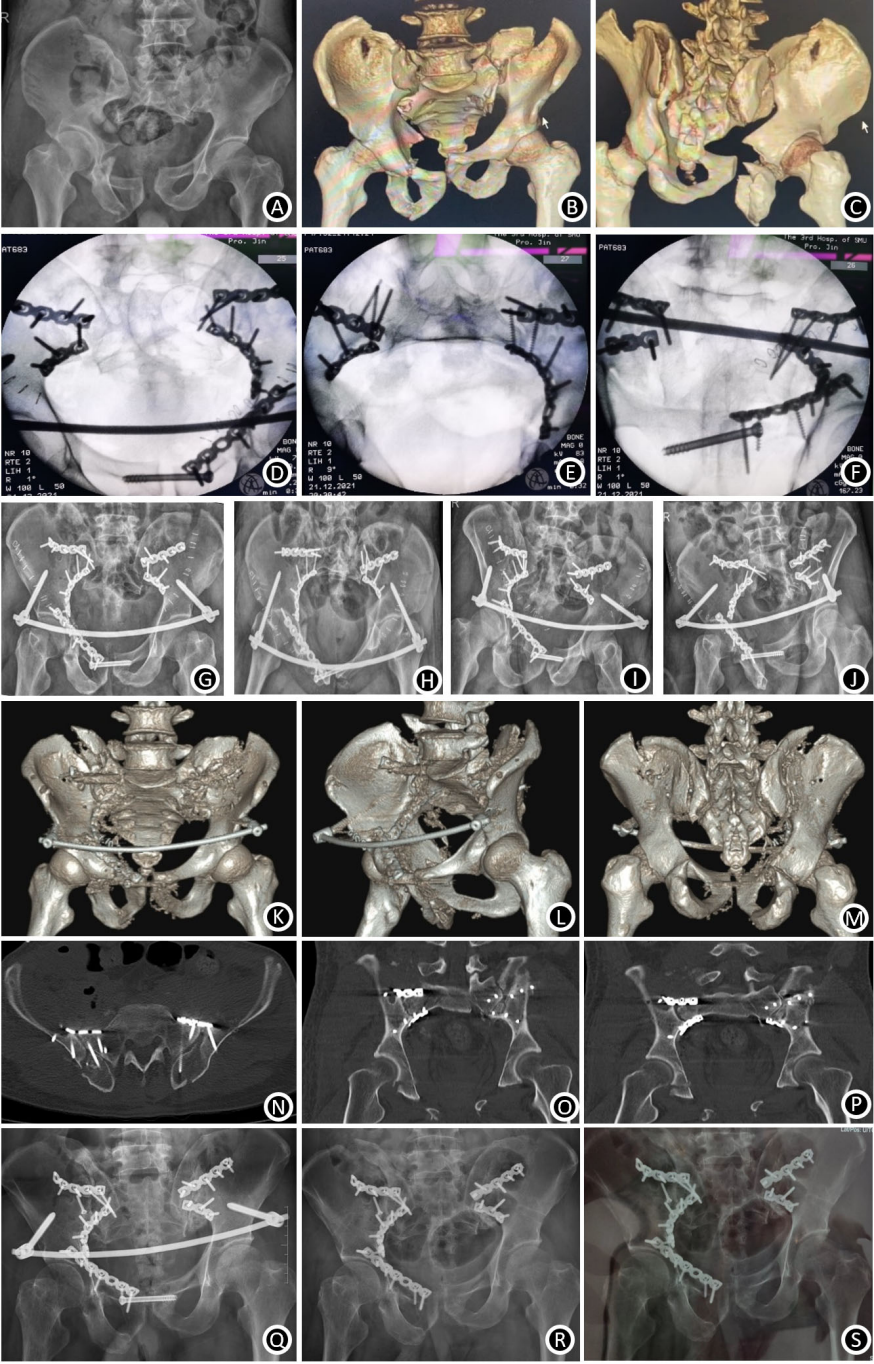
(A–C) Preoperative AP view and 3‐D reconstruction of CT data showed bilateral crescent‐shaped fractures of the posterior pelvic ring, anterior dislocation of the right sacroiliac joint, fractures of the superior and inferior pubic rami, and separation of the pubic symphysis; (D–F) AP, inlet and outlet views during operation: reduced the dislocated ilium by placing a plate on the sacroiliac joint and another plate on the arcuate line, and the anterior pelvic ring was fixed by a lag screw, an anterior column reconstructive plate and an internal fixator (INFIX); (G–M) Postoperative AP, inlet, obturator oblique, iliac oblique views, 3‐D reconstruction of CT data: ideal reduction and internal fixation was achieved; (N–P) Postoperative CT scan: the sacroiliac joint was well reduced and the angulated plates provided biplane stability for the joint; (Q) Postoperative AP view at 4‐month follow‐up before removing the INFIX frame; (R) Postoperative AP view at 4‐month follow‐up after removing the INFIX frame; (S) Postoperative AP view at 1‐year follow‐up: no fragments dislocation or loosening of internal fixation.
